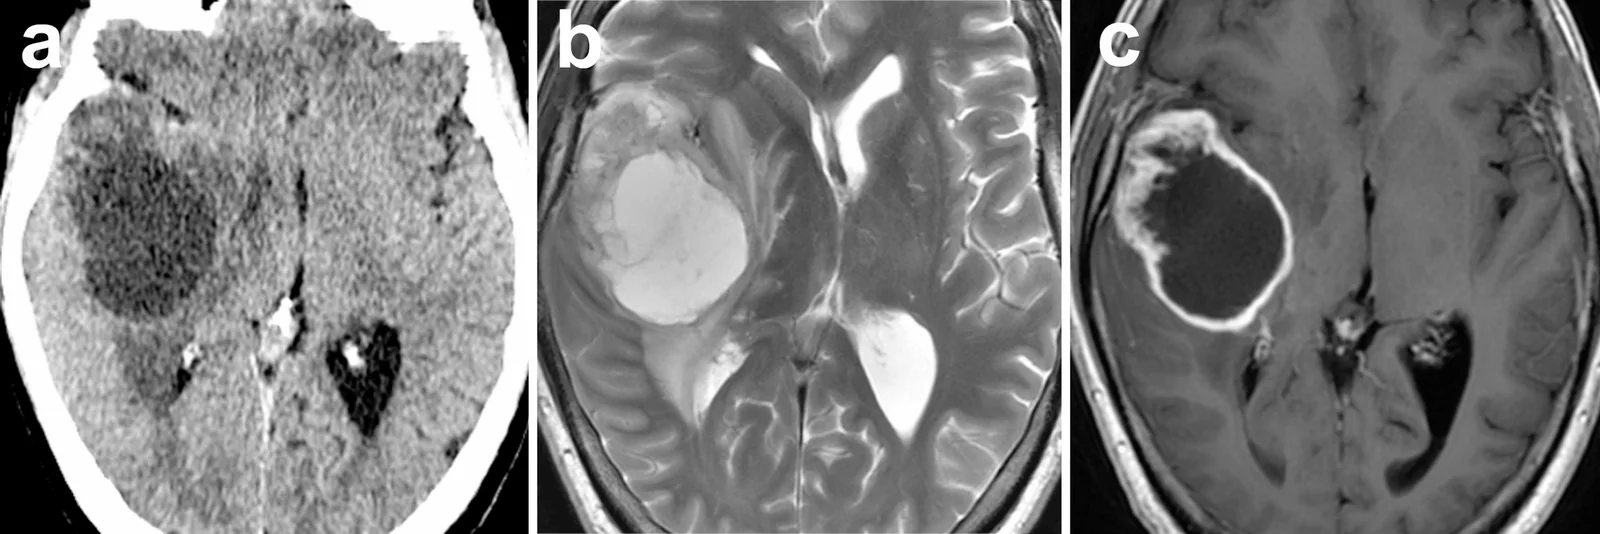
A 65-year-old man with glioblastoma presenting with headache. CT image (a) shows a cystic lesion in the right temporal lobe with surrounding edema and a midline shift to the left. On MRI, the T2-weighted image (b) demonstrates the cystic lesion, and the contrast-enhanced T1-weighted image (c) reveals irregular thick enhancement along the cyst wall
